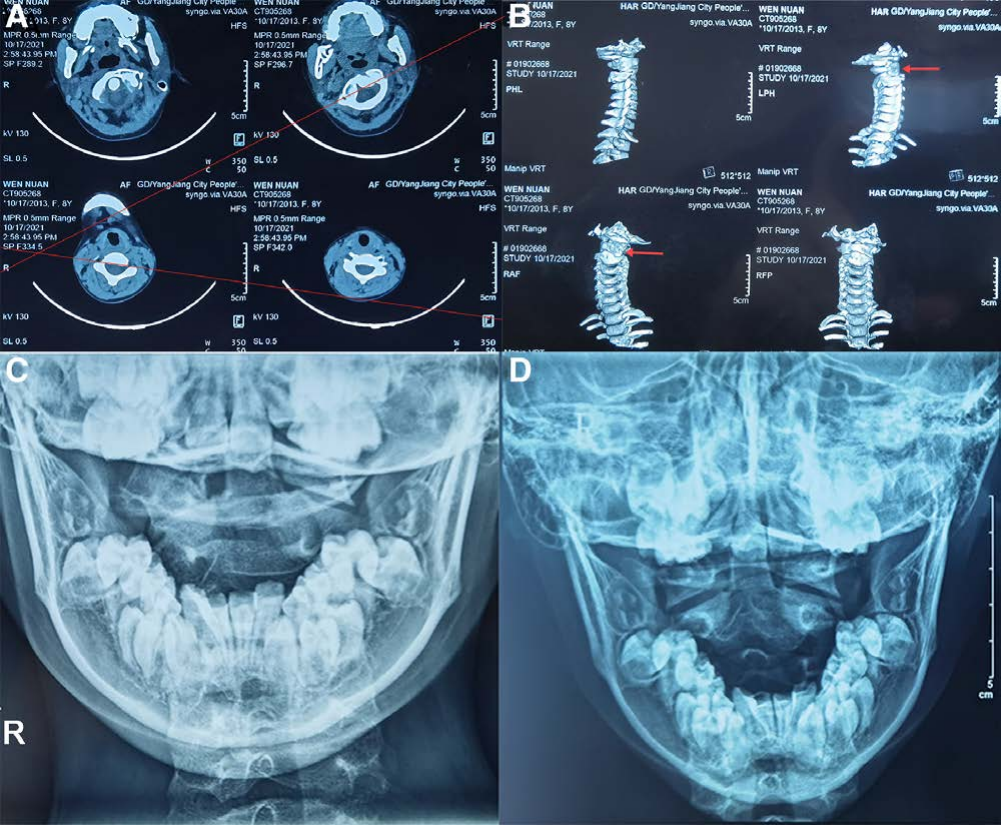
Three-dimensional CT of the cervical spine before massage treatment: (A) 3-dimensional CT of cervical spine (the angle between the 2 red lines is about 40 degrees); (B) 3-dimensional CT of cervical spine (red arrow refers to the site of rotation). X-ray of the open-mouth position cervical spine after massage treatment: (C) X-ray of the open-mouth position cervical spine (November 29, 2021 review); (D) X-ray of the open-mouth position cervical spine (December 08, 2021 review). CT = computed tomography.
